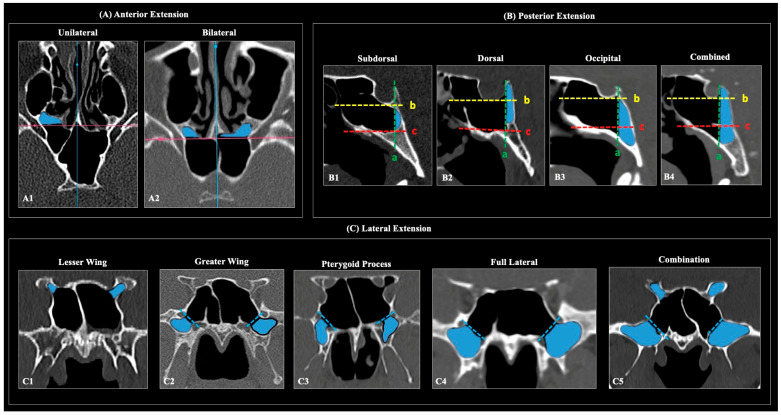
Patterns of sphenoid sinus pneumatization (SSP) on computed tomography (CT) according to anterior, posterior, and lateral extensions (blue colored). (A) Anterior extension: unilateral (A1) and bilateral (A2) pneumatization extending anteriorly beyond the anterior wall of the sphenoid sinus—SS (pink horizontal line). (B) Posterior (clival) extension: (B1) subdorsal—pneumatization extends posteriorly beyond the posterior wall of the sella (a, dotted green line) but remains between the line along the sellar floor (b, dotted yellow line) and the horizontal plane through the superior margins of the paired Vidian canals (c, dotted red line); (B2) dorsal—pneumatization extends above line (b); (B3) occipital—pneumatization extends below line (c); (B4) combined—pneumatization involves the entire clivus, extending both above (b) and below (c). Blue contours delineate the extent of pneumatization in each variant. (C) Lateral extension: pneumatization patterns involving (C1) the lesser wing—LW, (C2) greater wing—GW, (C3) pterygoid process—PP, (C4) full lateral—FL (GW+ PP), (C5) combined extension types.
